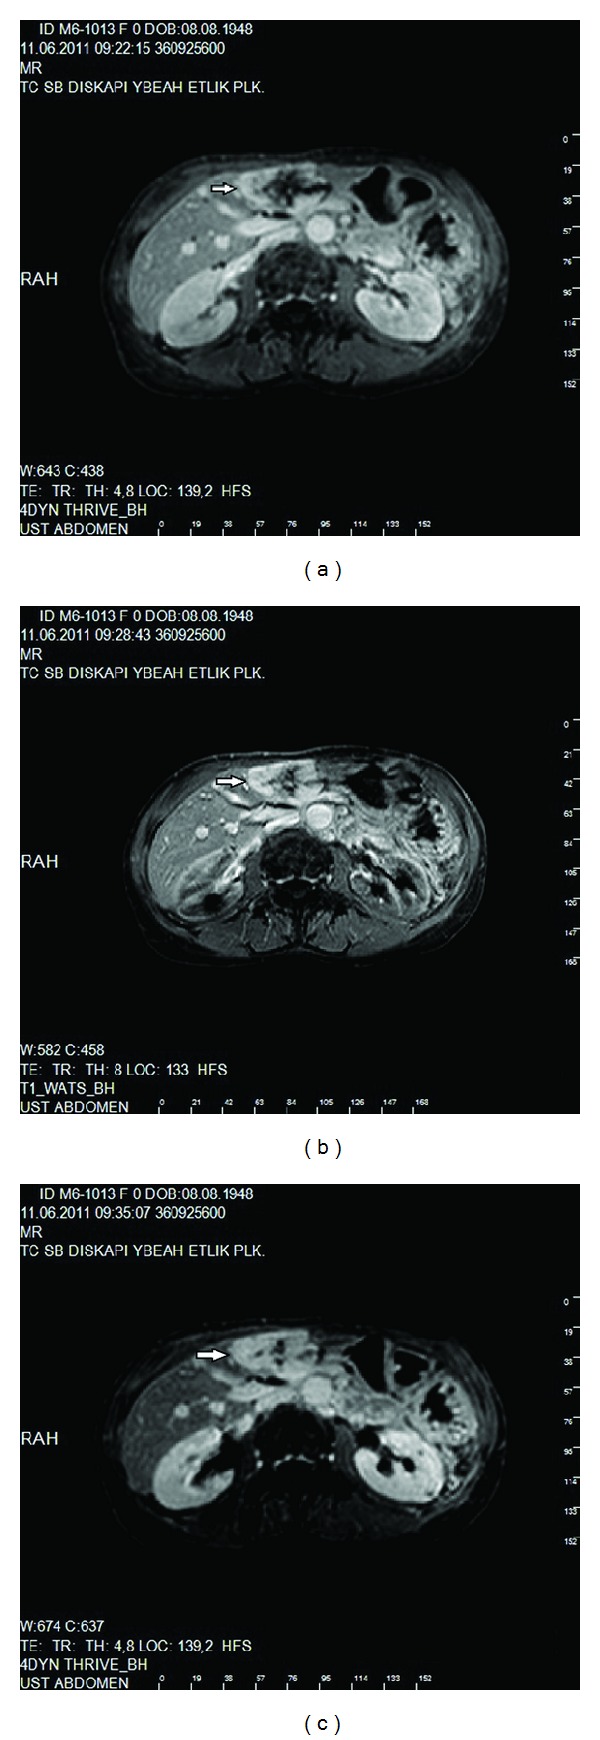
(a) On precontrast T1W images, a lobulated hyperintense liver mass in the left lobe with central-peripheral scar tissue (arrow). (b) On postcontrast early arterial MRI, the lesion showed hyperintense peripheral nodular enhancement (arrow). (c) On postcontrast delayed MRI, increasing central enhancement was seen in the lesion (arrow). The central-peripheral scar tissue did not show any enhancement on all images.
